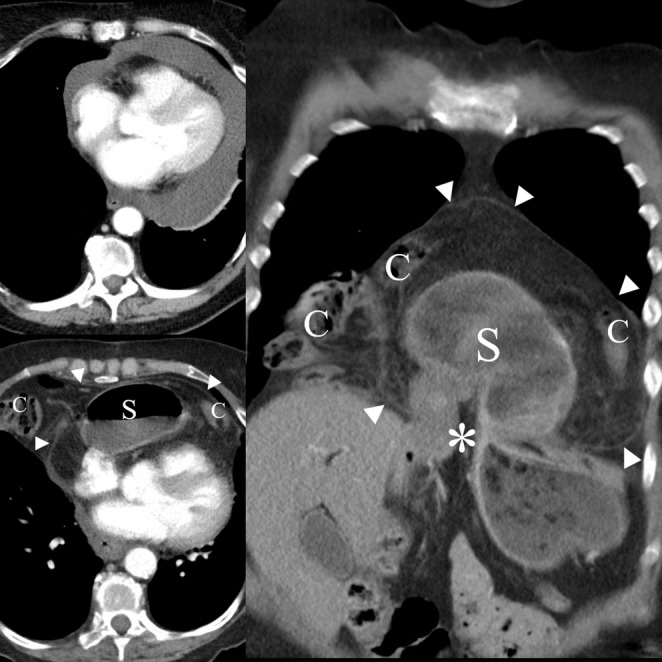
Contrast-Enhanced CT Imaging Before and After Pericardial Window Procedure. Top Left: Axial CT image before pericardial window procedure showing large effusion. Postoperative axial CT image (bottom left) and coronal CT image (right) demonstrate a diaphragmatic hernia (*) into the distended pericardium (arrowheads) at the surgical site, containing the distal stomach (S) as well as colon (C).
